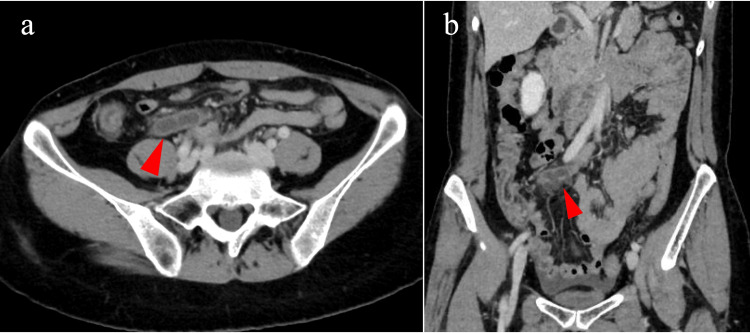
Enlarged appendix (red arrowhead) on contrast-enhanced CT of the abdomen. (a) Axial section. (b) Coronal section. CT, computed tomography
